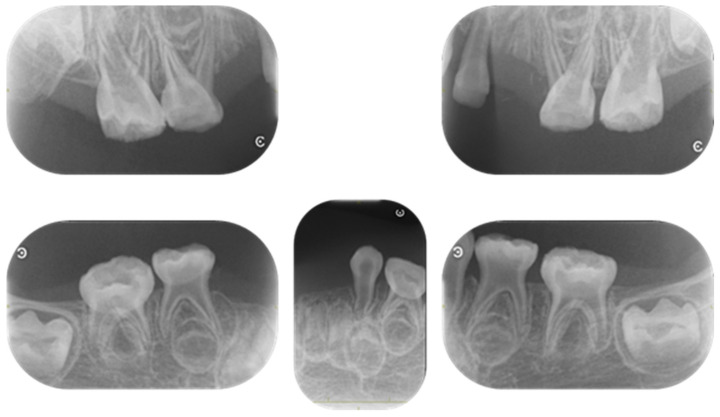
Periapical radiographs at the age of 3 years and 4 months.
